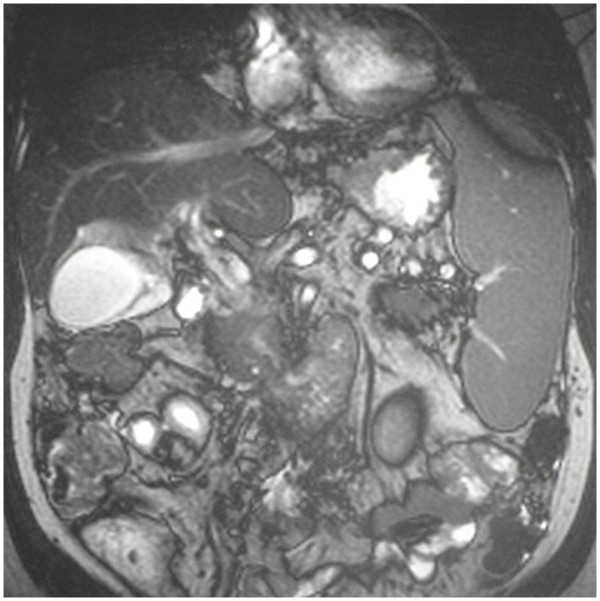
Magnetic resonance imaging of patient's abdomen shortly after admission. Saggital Magnetic Resonance Image showing varices around the gallbladder as well as marked splenomegaly.
